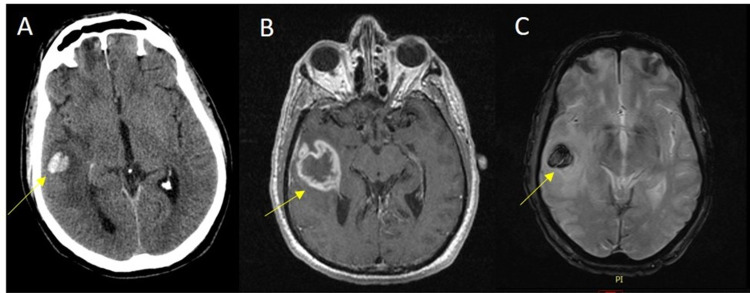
Axial noncontrast CT (A) demonstrates intraparenchymal presumed hematoma in this post-traumatic patient. Axial postcontrast T1 MRI image (B) shows a thick rim enhancing focus at the site of hemorrhage, which is confirmed by blooming on GRE series (C). There is surrounding edema. GRE: gradient recall echo.
